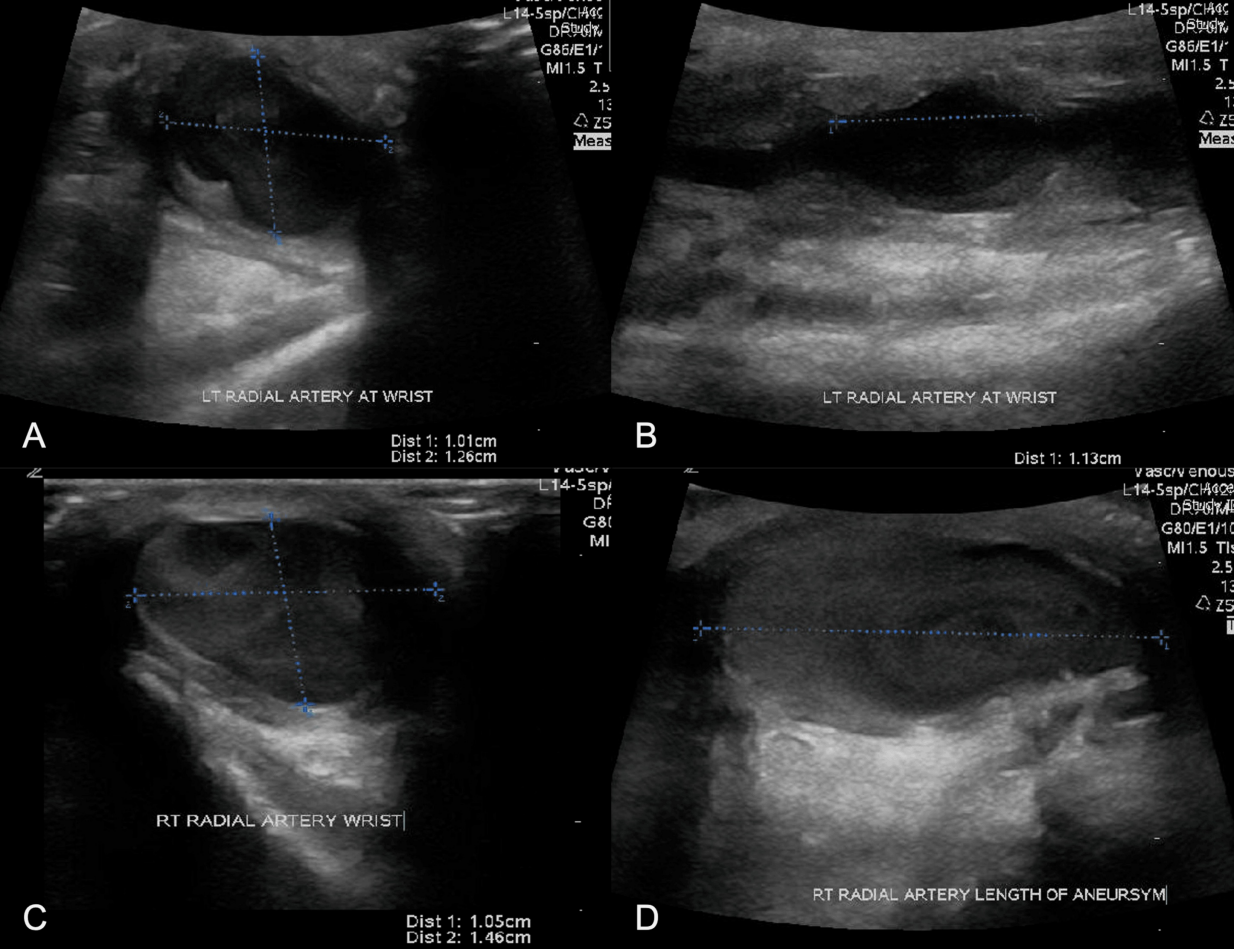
Doppler imaging of bilateral forearms. (A) Left wrist: Radial artery aneurysm measuring 1.01 cm in height and 1.26 cm in width. (B) Left wrist: Length of the radial artery aneurysm measuring 1.13 cm. (C) Right wrist: Radial artery aneurysm measuring 1.05 cm in height and 1.46 cm in width. (D) Right wrist: Length of the radial artery aneurysm measuring 2.57 cm.
